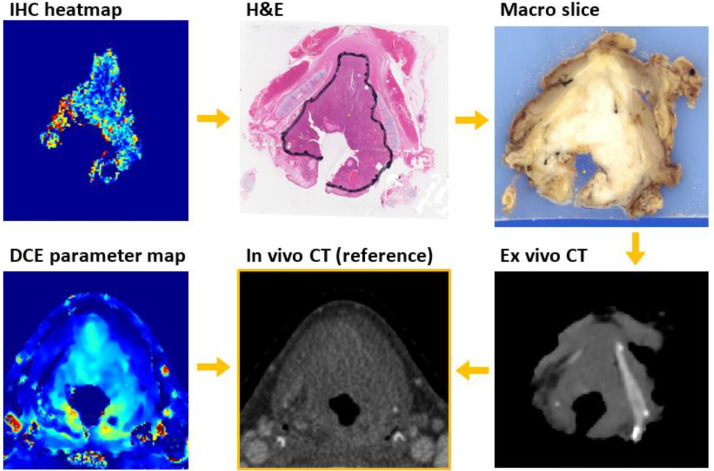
Registration procedure of IHC slices to the in vivo imaging. The in vivo CT is used as the reference image.
